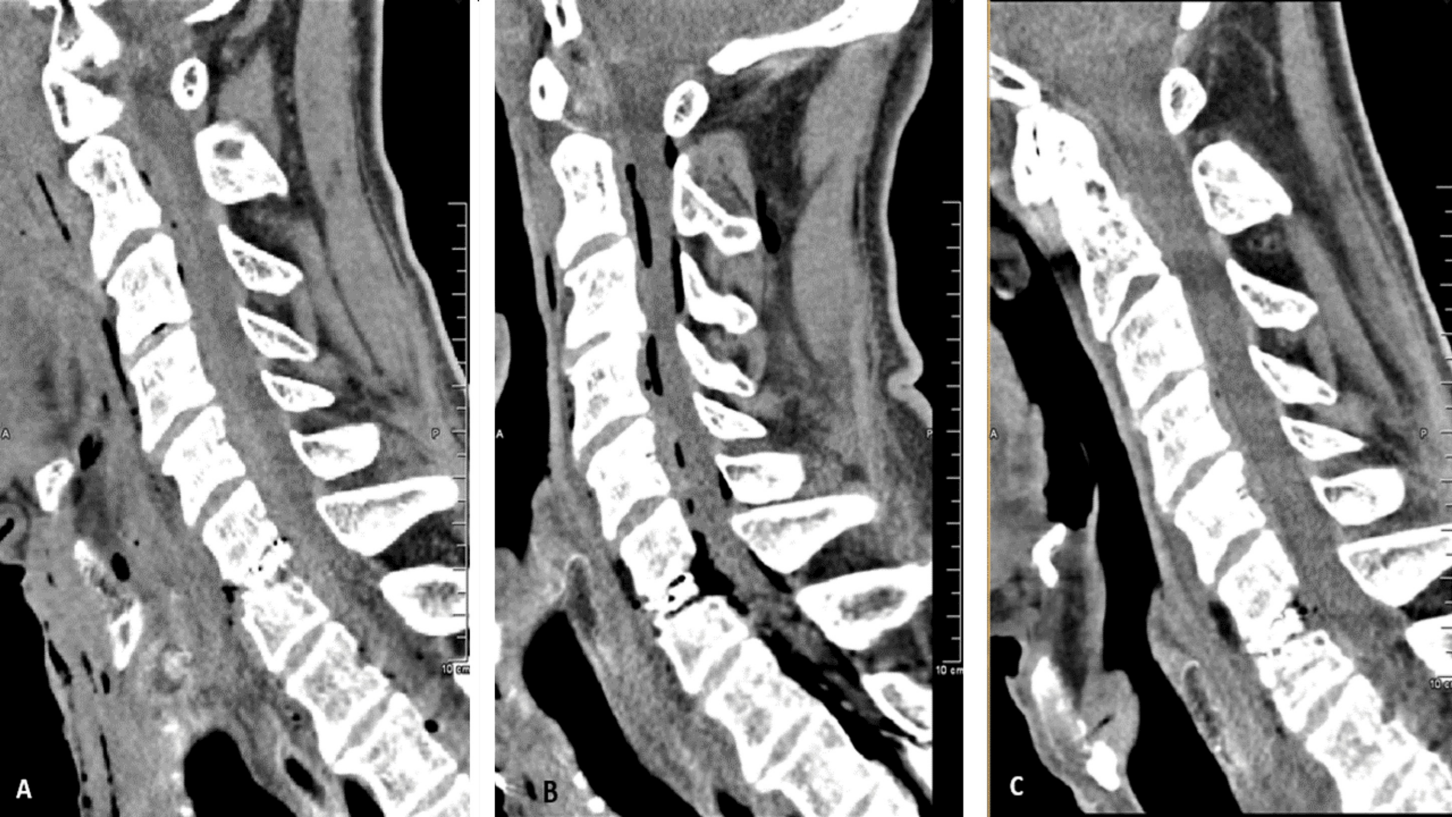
Sagittal CT imaging during the course of treatment: (A) Postoperative scan following ACDF showing an extensive epidural hematoma extending from C1 to T3. (B) Immediate postoperative scan after revision surgery demonstrating a small residual hematoma and postoperative air. (C) Follow-up scan five days after revision surgery confirming complete resolution of the hematoma. ACDF, anterior cervical discectomy and fusion
